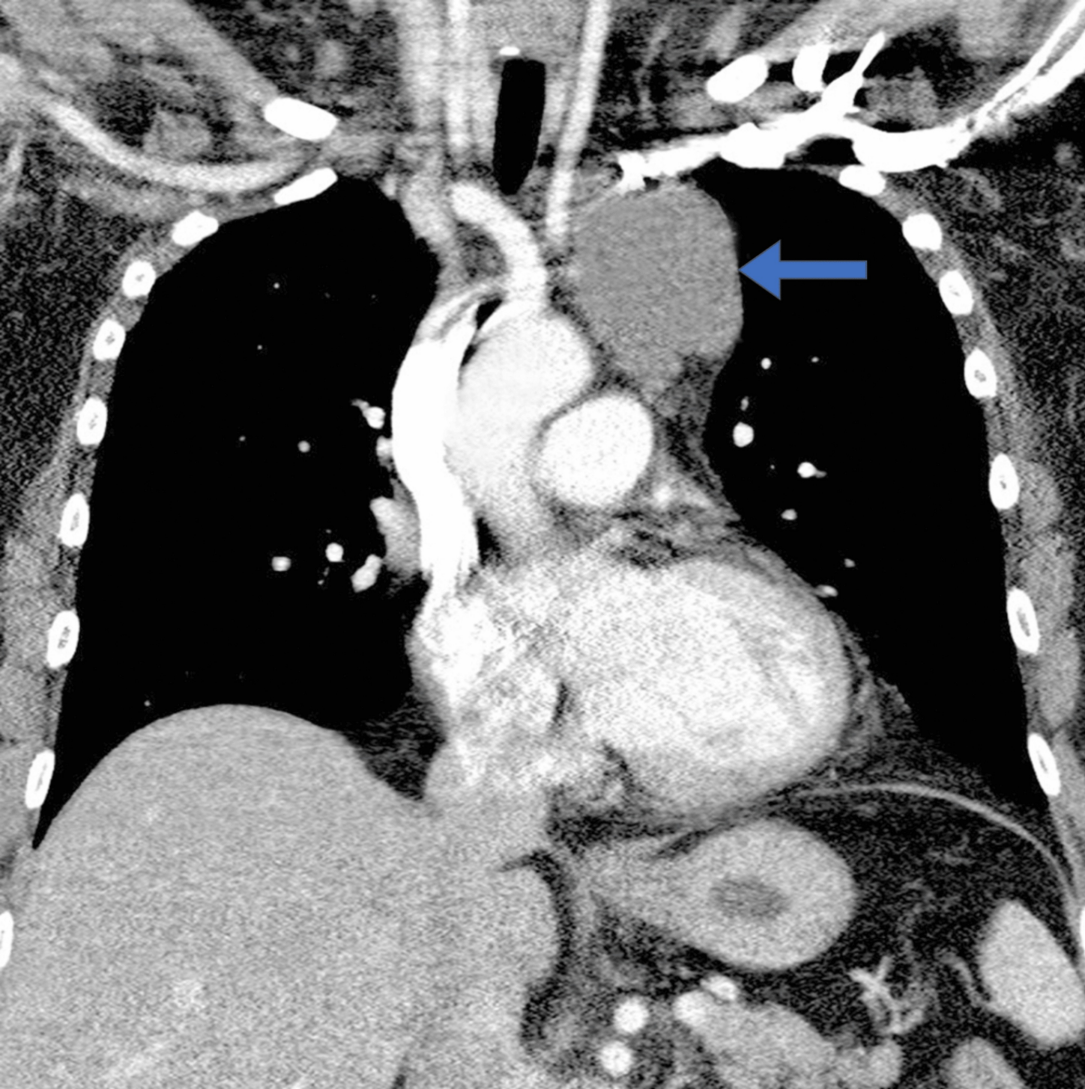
Coronal CT image demonstrates a lobulated cystic lesion (arrow) in the superior mediastinum
